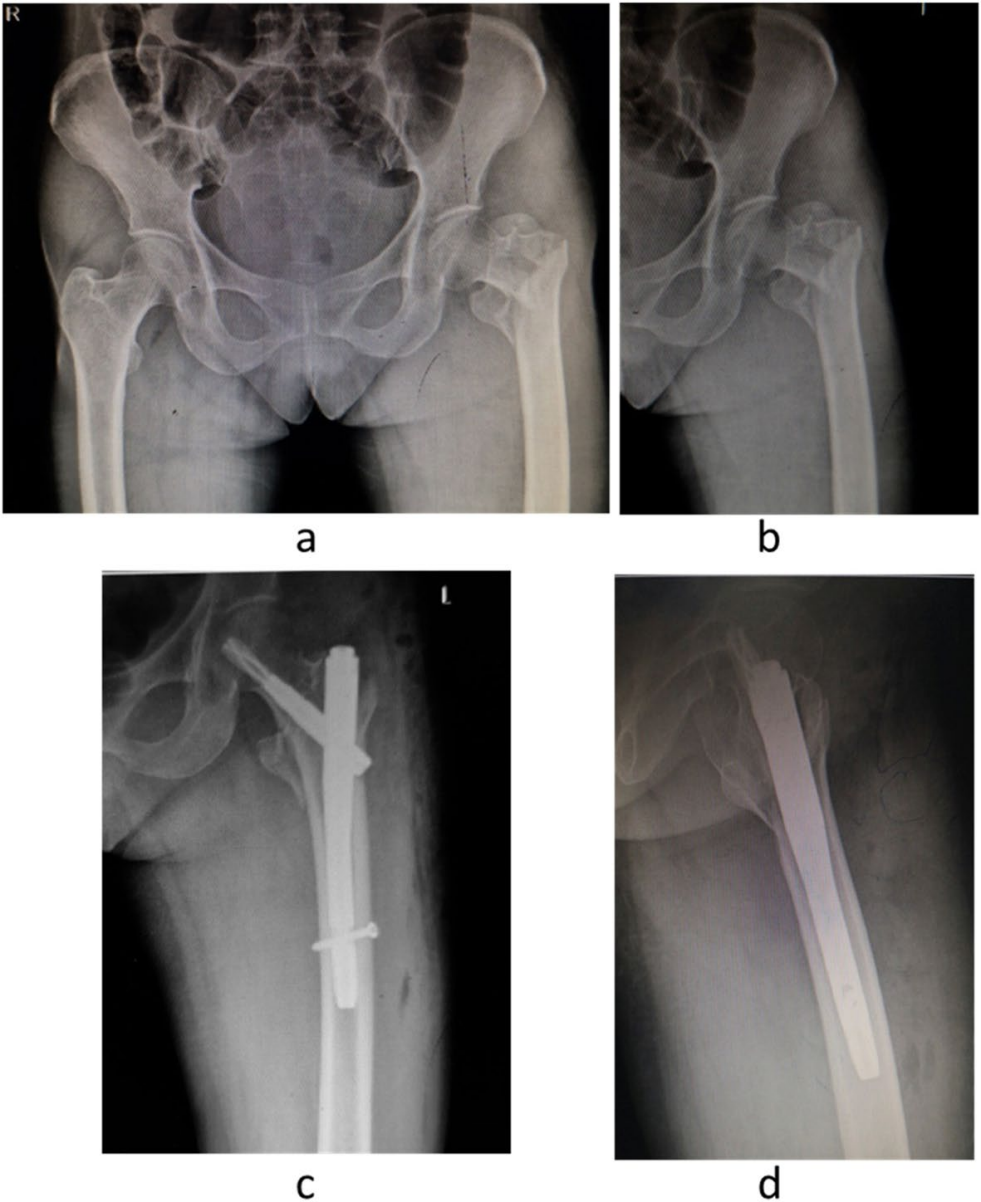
Anteroposterior radiograph showing an unstable intertrochanteric fracture of left hip in a 76-year-old female patient who fell at home (a, b). Anteroposterior pelvis and lateral femoral examination after the operation showed good fracture alignment and satisfactory fixation (c, d)
